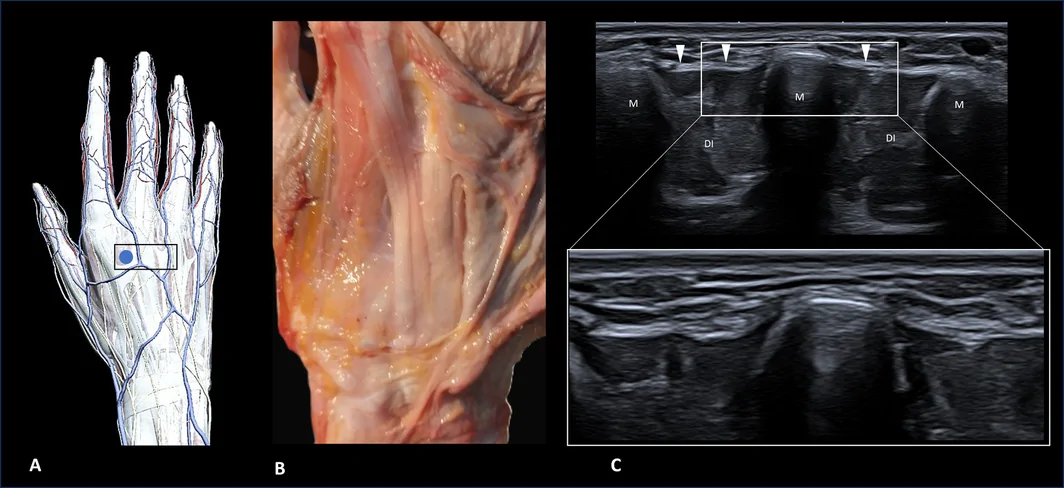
Dorsal fascia of the hand. A, Probe positioning. B, Anatomical dissection showing the dorsal fascia of the hand, appearing as epimysial layers, often less distinct from underlying muscles. C, Ultrasound images revealing the dorsal fascia of the hand (arrowheads) as a thin hyperechoic band. M, Metacarpal bone; DI, dorsal interossei muscles.
